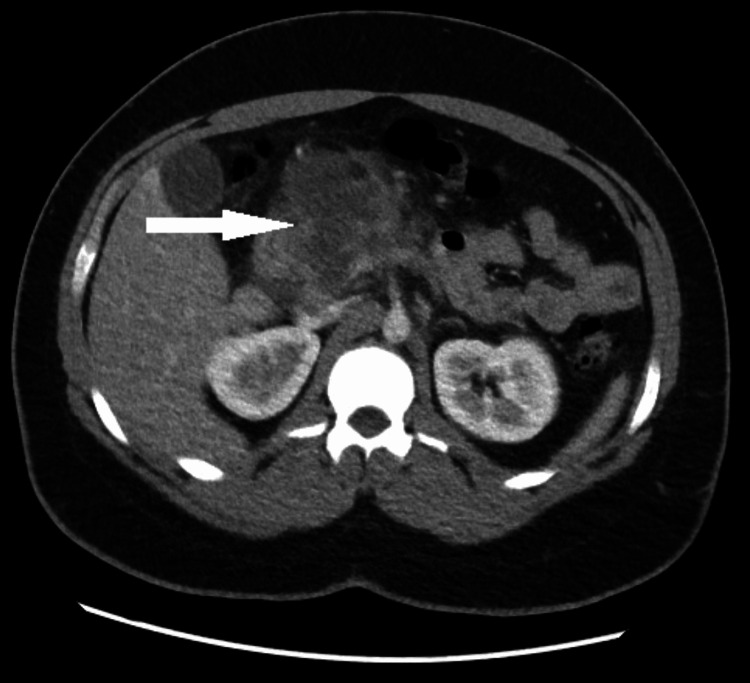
CT scan of the abdomen showing extensive necrosis of the head of the pancreas with peripancreatic fluid collection CT: computed tomography
